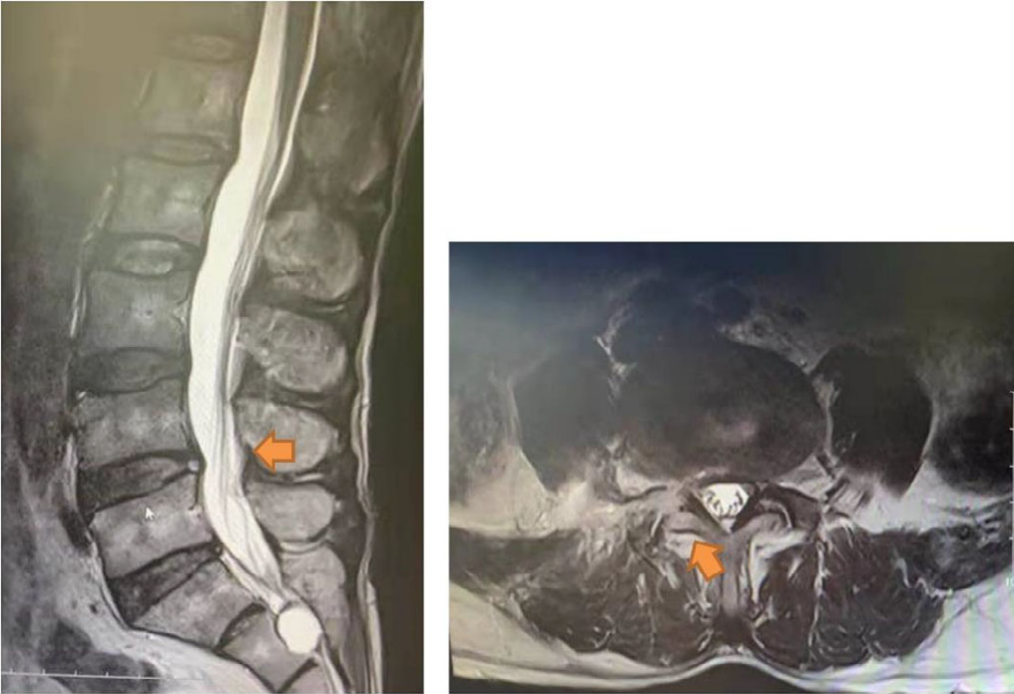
A postoperative MRI confirmed that the disc herniation was successfully decompressed, foraminoplasty can also be seen in the postoperative MRI.
